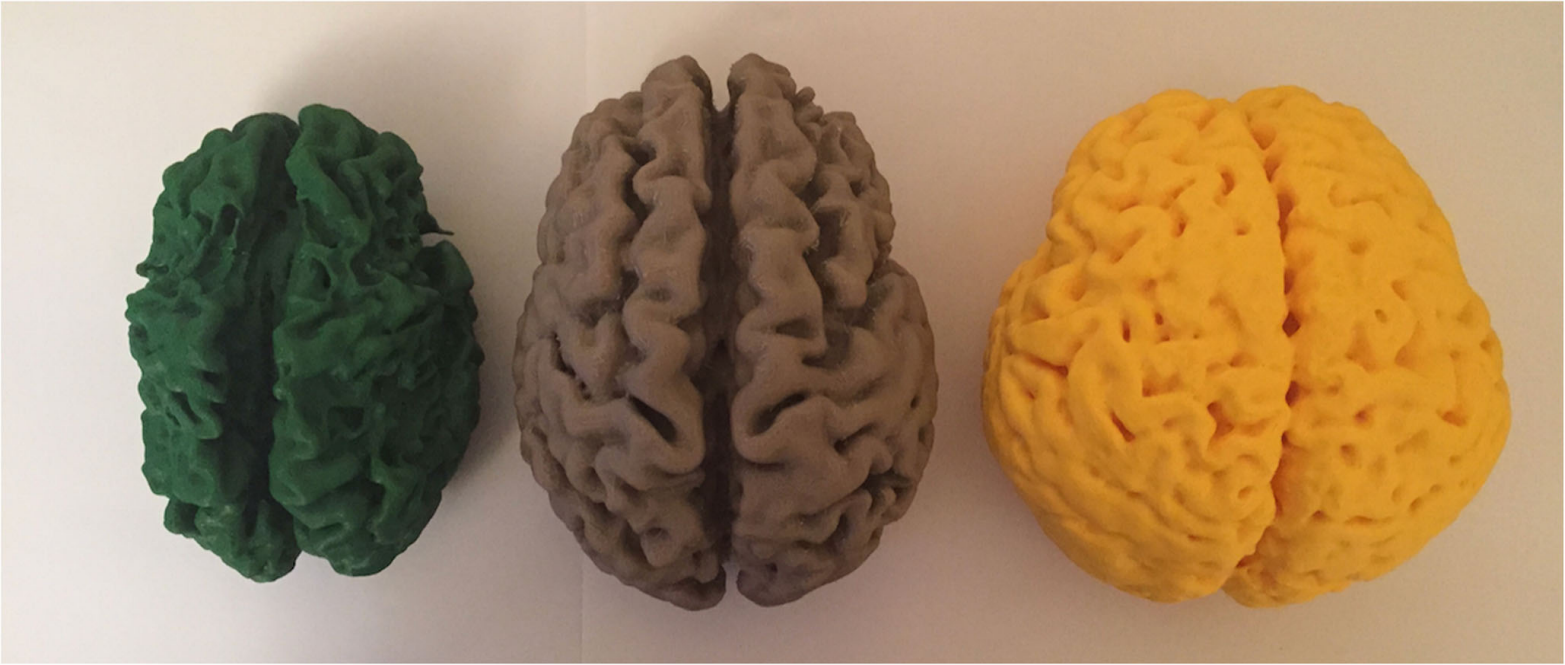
3D printed models of the brains of age matched children. On the left, a child severely affected by partial-prolonged hypoxic ischaemic injury [GREEN brain]; in the middle, a child with mild/moderate hypoxic ischaemic injury predominantly affecting the parasagittal and anterior watershed zones [GREY brain]; on the left a healthy brain [YELLOW brain]. When printed at scale and placed side-by-side, the extent of volume loss due to atrophy is far more apparent than when viewing axial images on an MRI because they allow appreciation of scale
